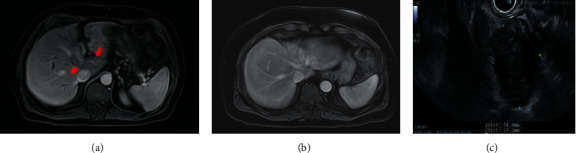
(a) Magnetic resonance imaging (MRI) of the abdomen demonstrating hepatic IPT prior to treatment with steroid taper. (b) MRI of the abdomen after treatment showing complete resolution of hepatic IPT. (c) Endoscopic ultrasound demonstrating a periportal mass.
